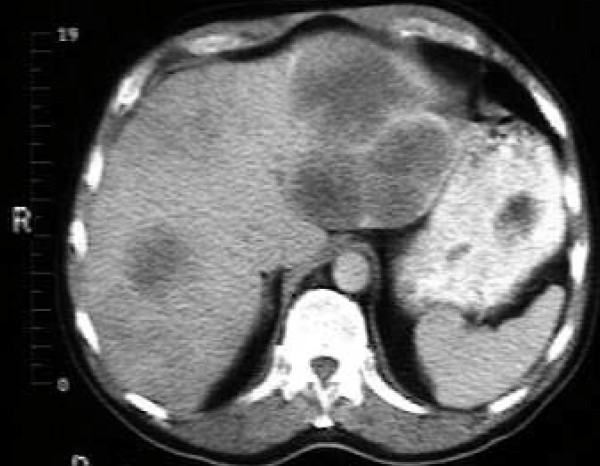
CT of her abdomen revealing multiple low density opacities in the liver consistent with metastases.
